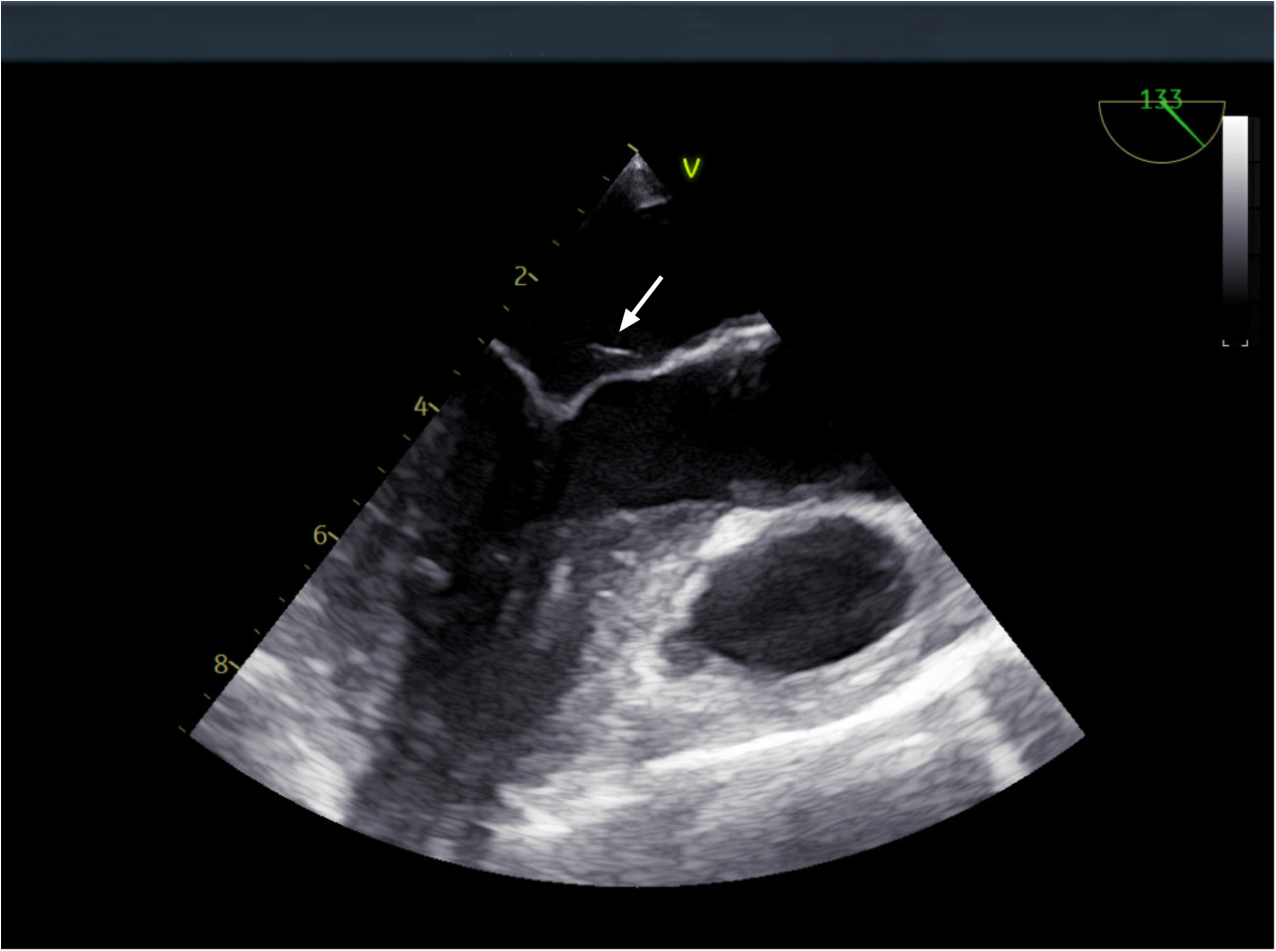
Transesophageal echocardiography: a vegetation on the mitral valve was detected. The arrow indicates the location of the mitral valve vegetation.
